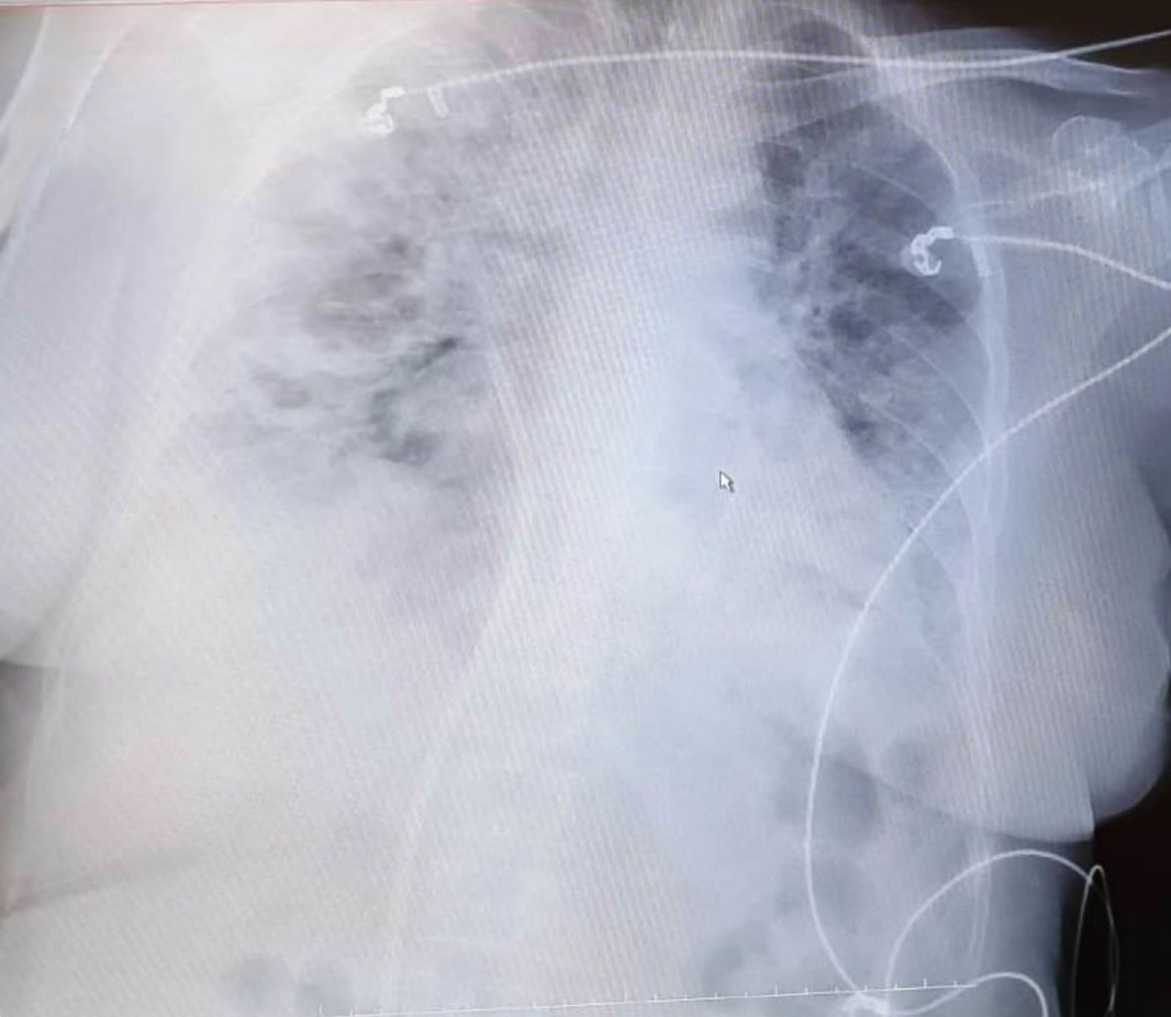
Chest X-ray revealed widespread bilateral alveolo-interstitial infiltrates.
